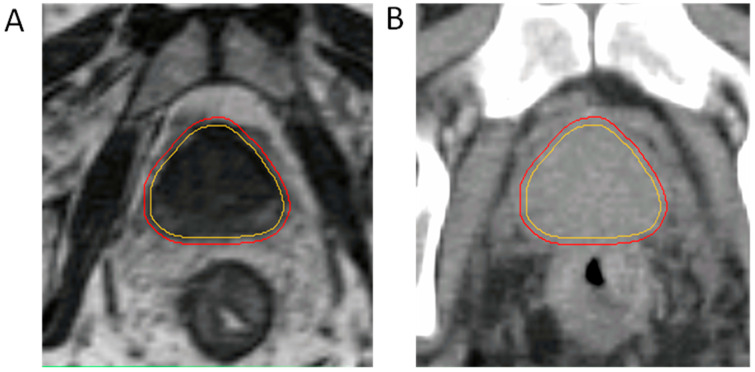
MRI-Linac-based contours on (A) the 0.35 Tesla MRIdian system and (B) a legacy CT planning scan. CTV is in yellow, isotropic 2 mm PTV is in red. Anatomic boundaries of the prostate are more clearly visualized with MRI guidance, enabling radical isotropic margin reduction from 4 mm to 2 mm. CT-only-based planning would result in a larger PTV margin than is currently displayed in image B.
